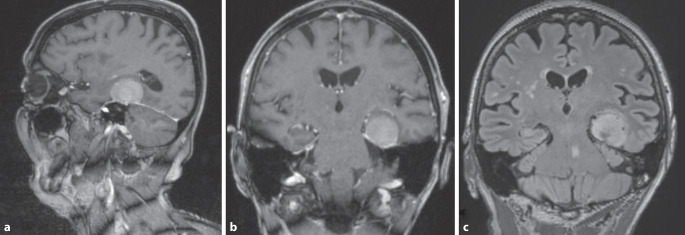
Magnetresonanztomographie des Kopfes: a sagittale, b koronar reformatierte MP-RAGE-Sequenz nach Kontrastmittelgabe, c koronar reformatierte FLAIR-Sequenz. Großes links temporobasales Meningeom mit Verlagerung und Kompression des linken Hippokampus
